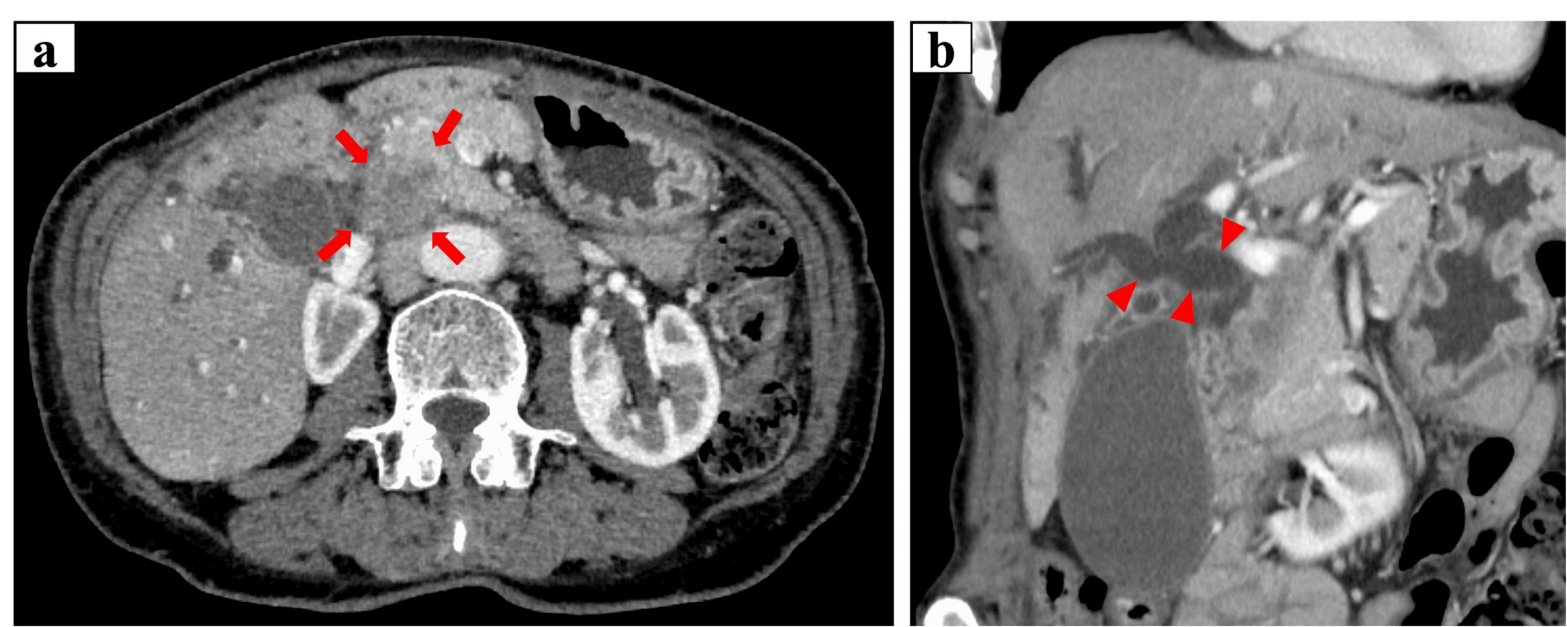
Contrast-enhanced abdominal CT at the initial diagnosis showing (a) a mass located in the head of the pancreas (arrows), and (b) moderate dilation of the common bile duct and intrahepatic bile ducts (arrow heads).
